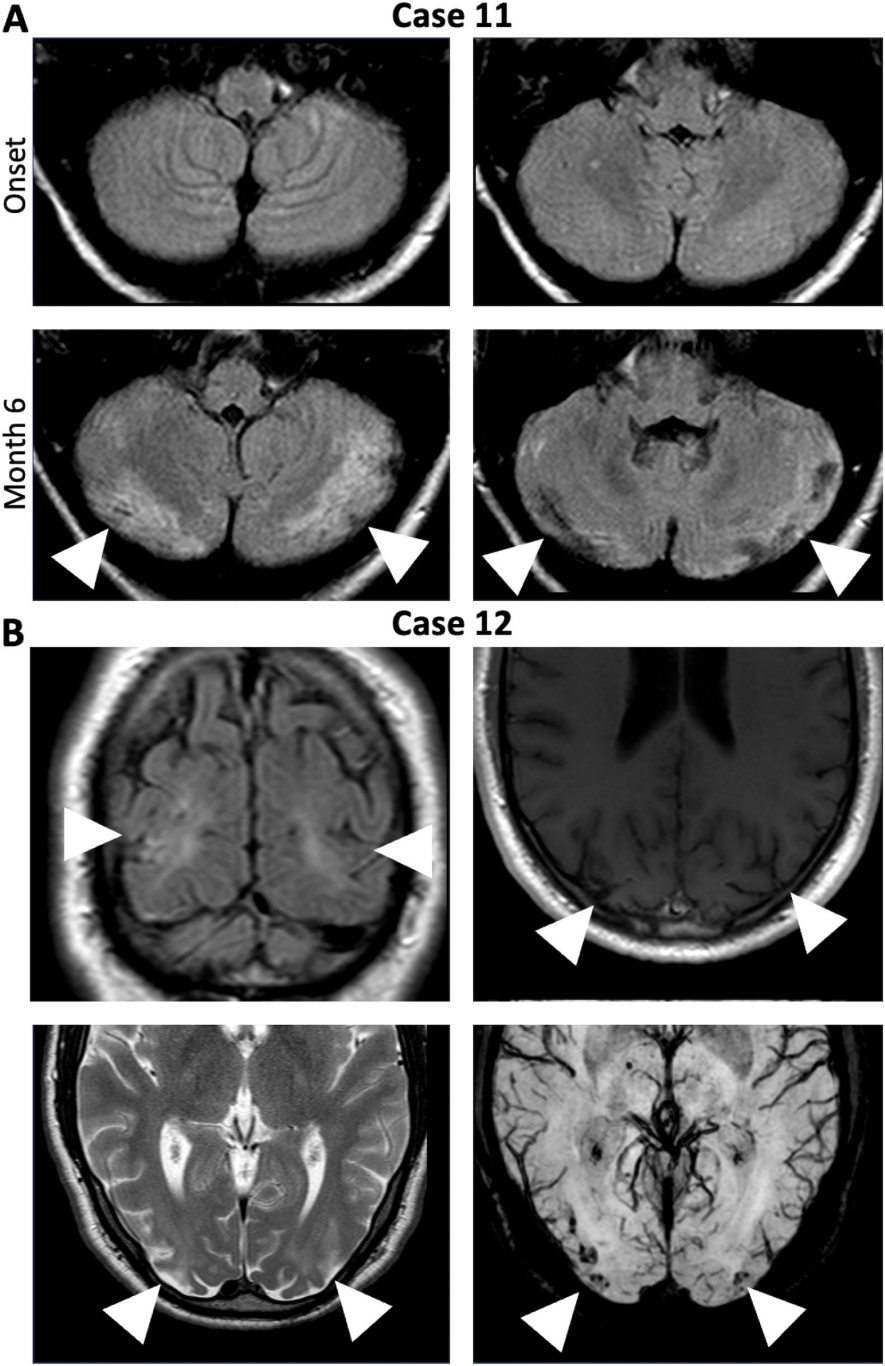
Symptomatic non-cerebellar and cerebellar ataxia due to ischemic hypoxic lesion acquired during circulatory failures in severe NMDAR-E. (A) Cerebral FLAIR MRI of Case 11 at onset did not show any cerebellar abnormalities (upper panels). After resuscitated 6 months after disease onset (lower panel, Month 6), repeat cerebral MRI showed bilateral subacute cerebellar infarctions (lower panels, arrowheads). (B) Bilateral hemodynamic ischemic lesions in the parietooccipital region (arrowheads) with hemorrhagic transformation (left upper panel: coronal FLAIR, right upper panel: transverse T1-weighted image, left lower panel: transversal T2-weighted image, right lower panel: Transverse susceptibility-weighted images (white arrows) in Case 12 after cardiopulmonary arrest and successful cardiopulmonary resuscitation.
